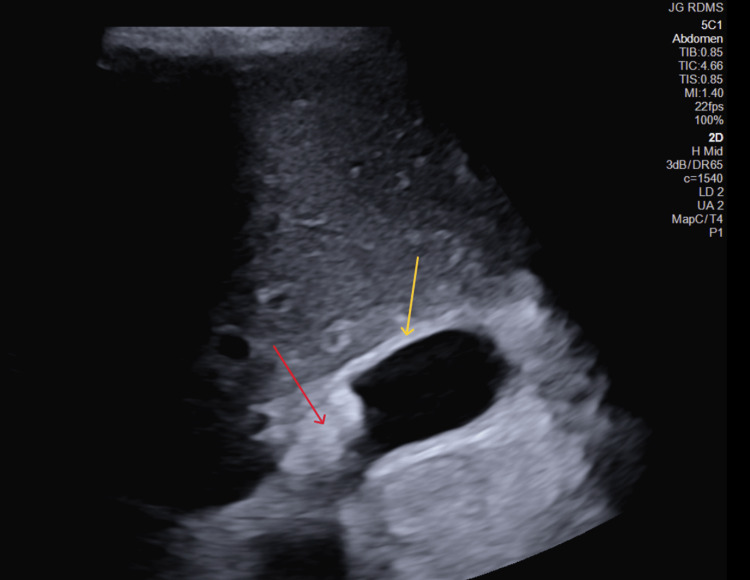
Abdominal ultrasound Red arrow: mobile cholelithiasis; yellow arrow: gallbladder wall thickening measuring >3 mm
